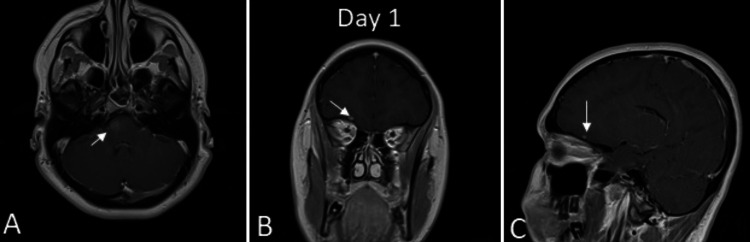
Initial MRIs of the patient (A) The anterior midline and anterior right side of the medulla oblongata-bulbus junction, which shows patchy gadolinium enhancement. (B,C) The basal area of the right frontal lobe, with a diameter of 5 mm, which shows patchy gadolinium enhancement MRI: magnetic resonance imaging
